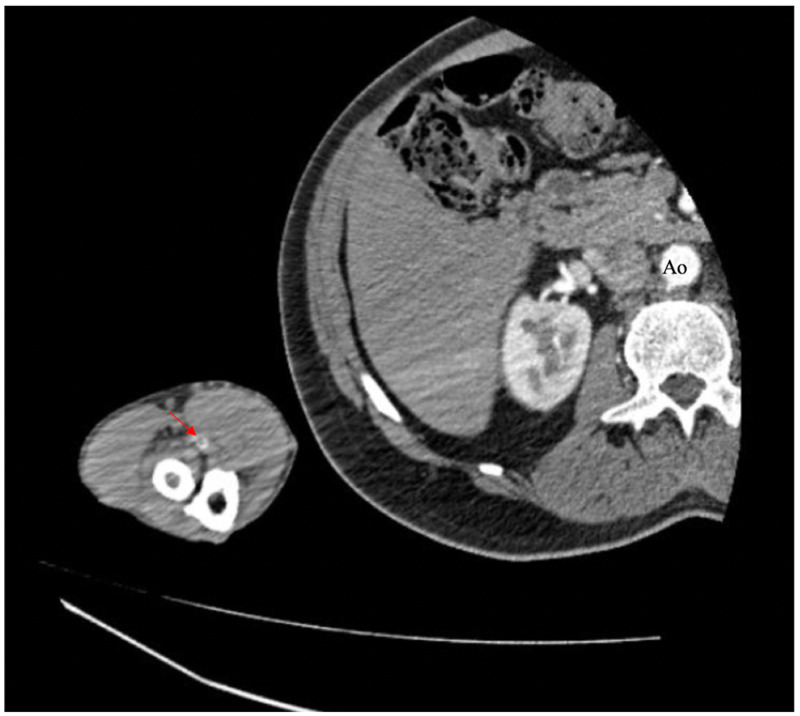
Computed tomography angiography (CTA) of the right-hand revealing thrombus formation (red arrow) and filling defect as compared to the aorta (Ao)
